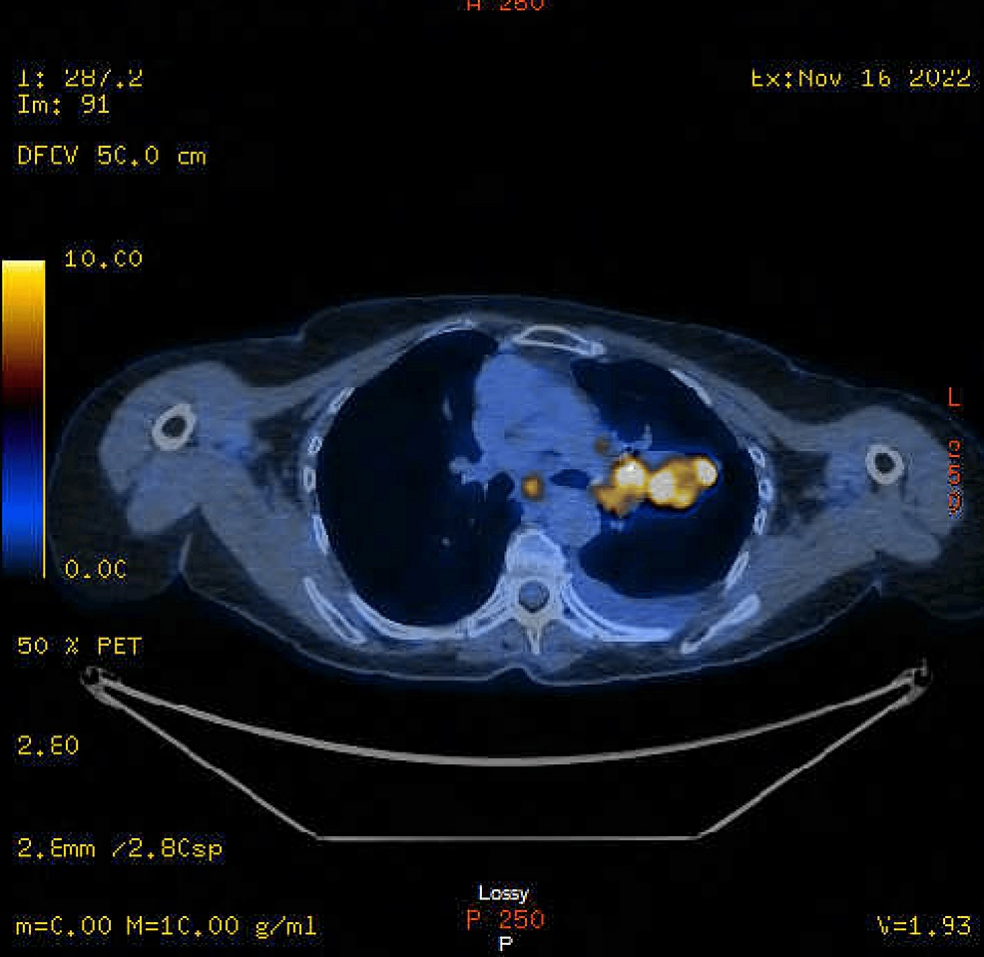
FDG-PET showing the left lung mass. FDG-PET: Fluorodeoxyglucose Positron Emission Tomography.
